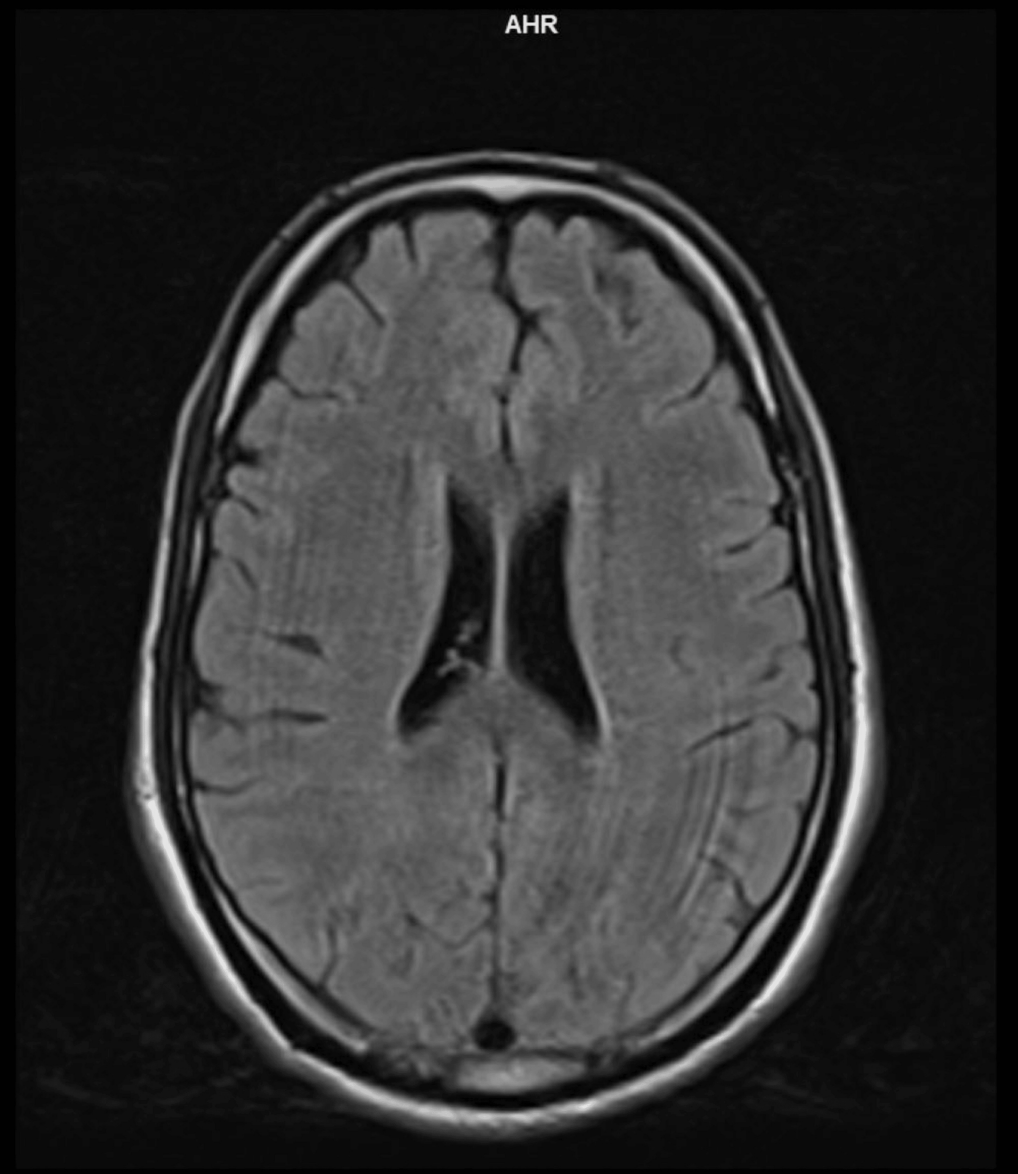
T2 FLAIR axial section showing no involvement of the cortex FLAIR: fluid-attenuated inversion recovery
